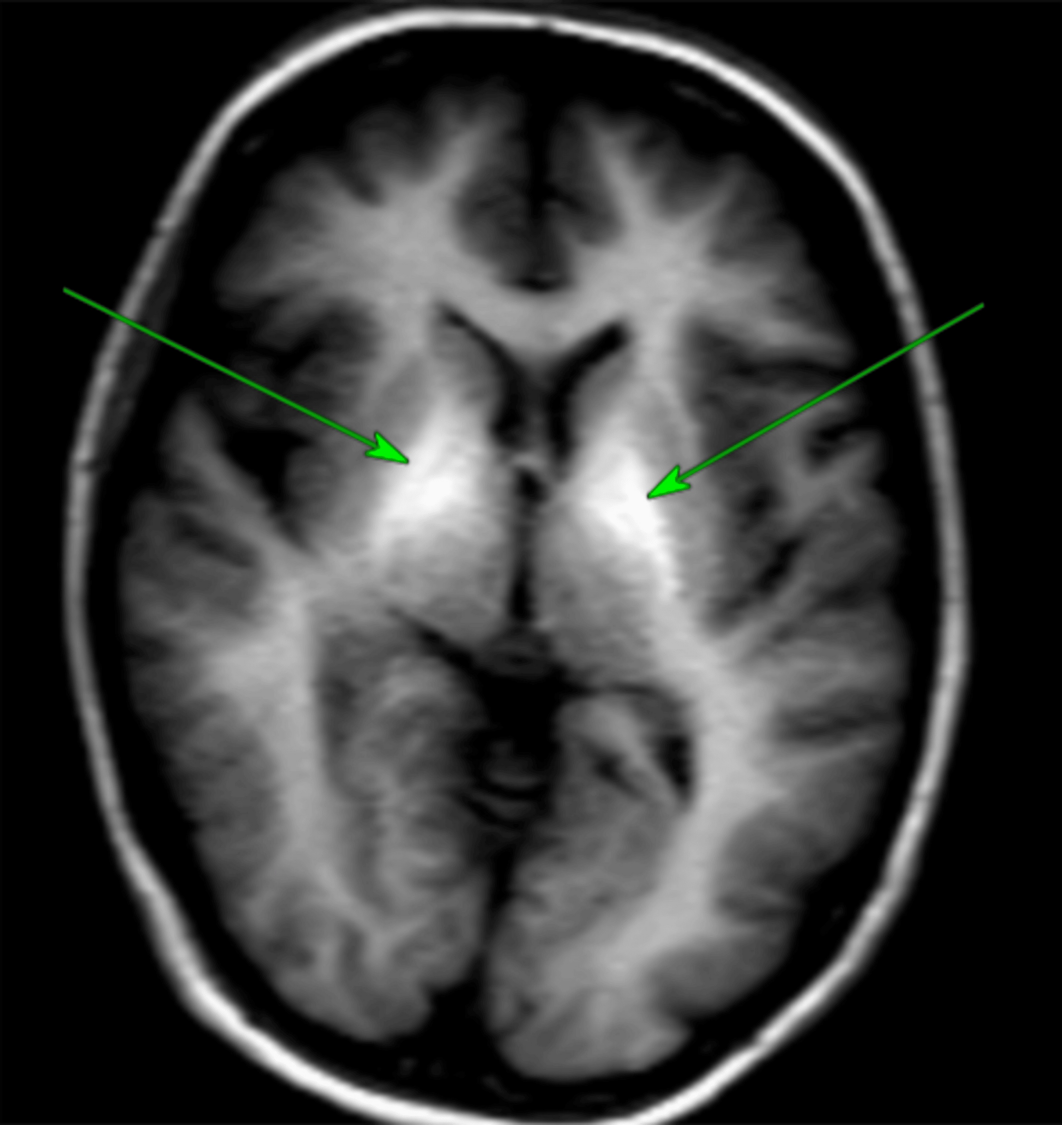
T1-weighted axial MRI of the brain The two arrows indicate the areas showing bilateral symmetric T1 hyperintensity in the globus pallidus
